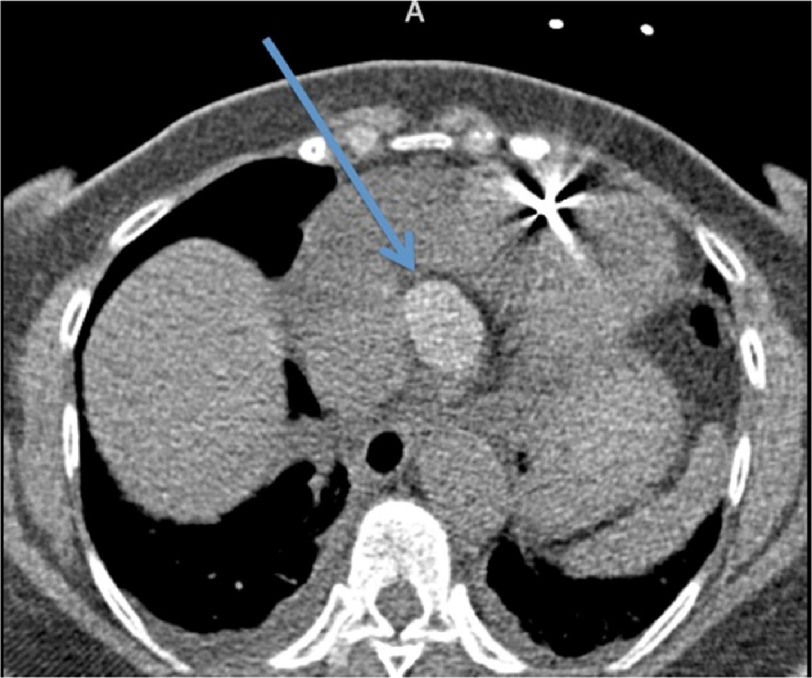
The mass was incidentally noted on an outpatient cardiac computerized tomography (CT) scan for calcium scoring. The arrow points to the 3.7 × 2.5 cm well-circumscribed, hyperdense mass on this axial cut.
